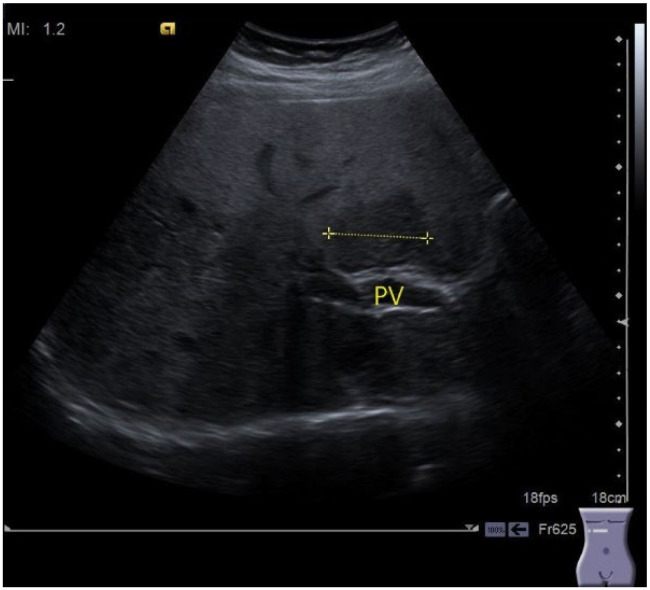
A 43 year-old obese female (BMI 32 kg/m^2^) presents for consultation for routine US examination. Ultrasound revealed a large hypoechoic area in segments VII, VIII with clear linear delineation from the rest of the liver. Just anterior to the portal vein (PV) another hypoechoic clearly delineated lesion (between markers +). Liver function tests normal, elevated triglycerides and glycemia, normal values of liver stiffness by 2D-SWE elastography. Typical aspect of focal fatty sparing.
